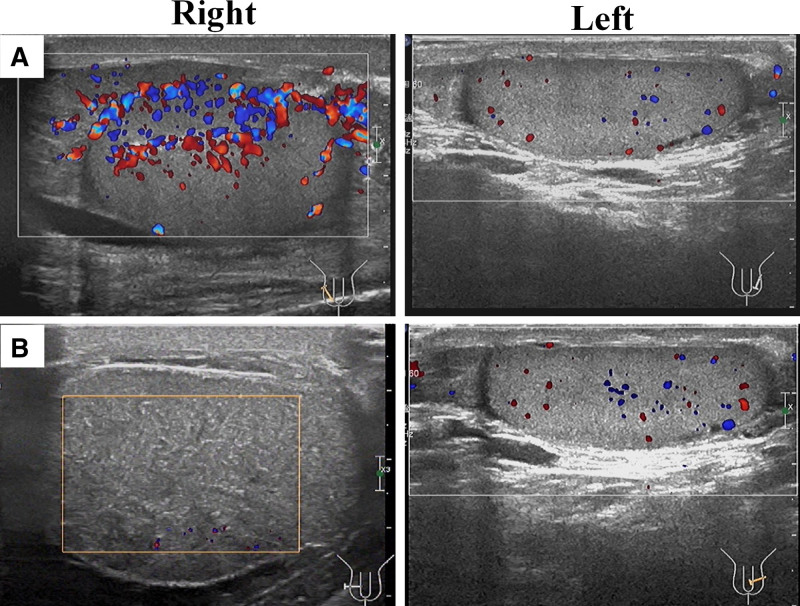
Scrotal ultrasound images in a 70-yr-old patient with scrotal pain. (A) Initial color Doppler ultrasonography reveals markedly increased blood flow in the right testis as compared to the physiological perfusion patterns in the left testis. (B) Day-11 follow-up ultrasound reveals disappeared perfusion in the right testis relative to the normal vascularity in the left testis.
